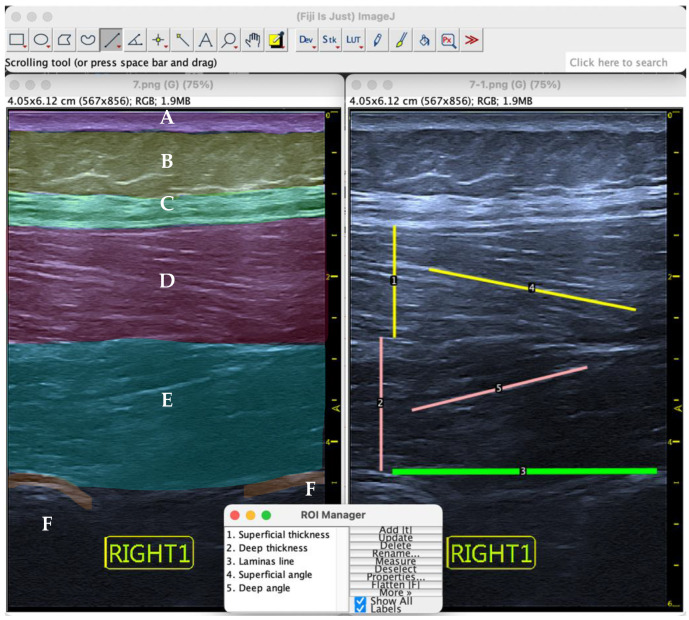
Ultrasound image of the lumbar multifidus musculature (sagittal view) during measurement of the thickness (lines 1–2) and pennation angle (lines 4–5). (A) Skin; (B) fat; (C) thoraco-lumbar fascia; (D) superficial multifidus; (E) deep multifidus; (F) laminae.
